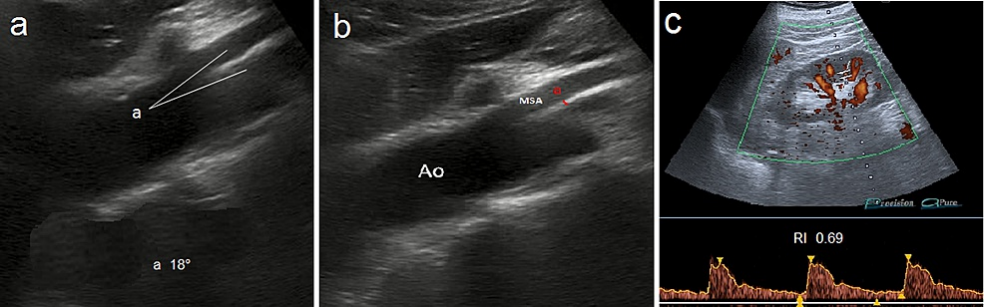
Images showing ultrasound examination (a) Longitudinal scan of abdominal aorta and MSA with aorto-mesenteric angle (a) measurement (18 degrees). (b) Aorto-mesenteric distance (a) measurement (6 mm). (c) Color Doppler US of left kidney shows regular Resistive Index (0.69).
